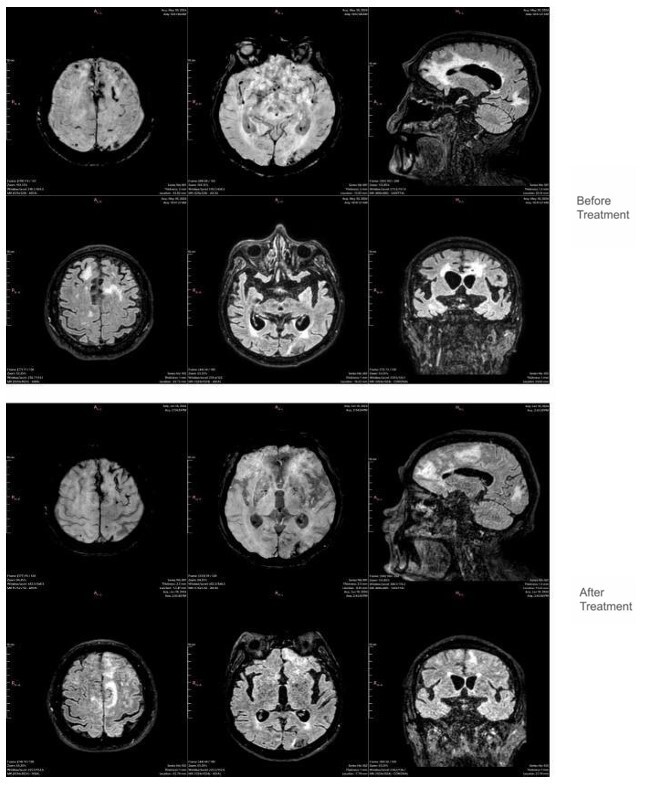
Images related to Case 1 include susceptibility-weighted imaging sequences (the first two figures in the top row) and their corresponding FLAIR sequences (the two figures immediately below). These demonstrate that the hyperintense signals in the FLAIR sequence correspond to areas with the highest concentration of hypointense signals on the susceptibility-weighted imaging sequence, which, in this context, are suggestive of hemorrhage. The rightmost image in the top row shows a sagittal FLAIR sequence, while the rightmost image in the second row displays a coronal FLAIR sequence, providing a better understanding of the extent of inflammation. Post-treatment images, shown in the two bottom rows, represent the same anatomical locations as those in the top rows.
